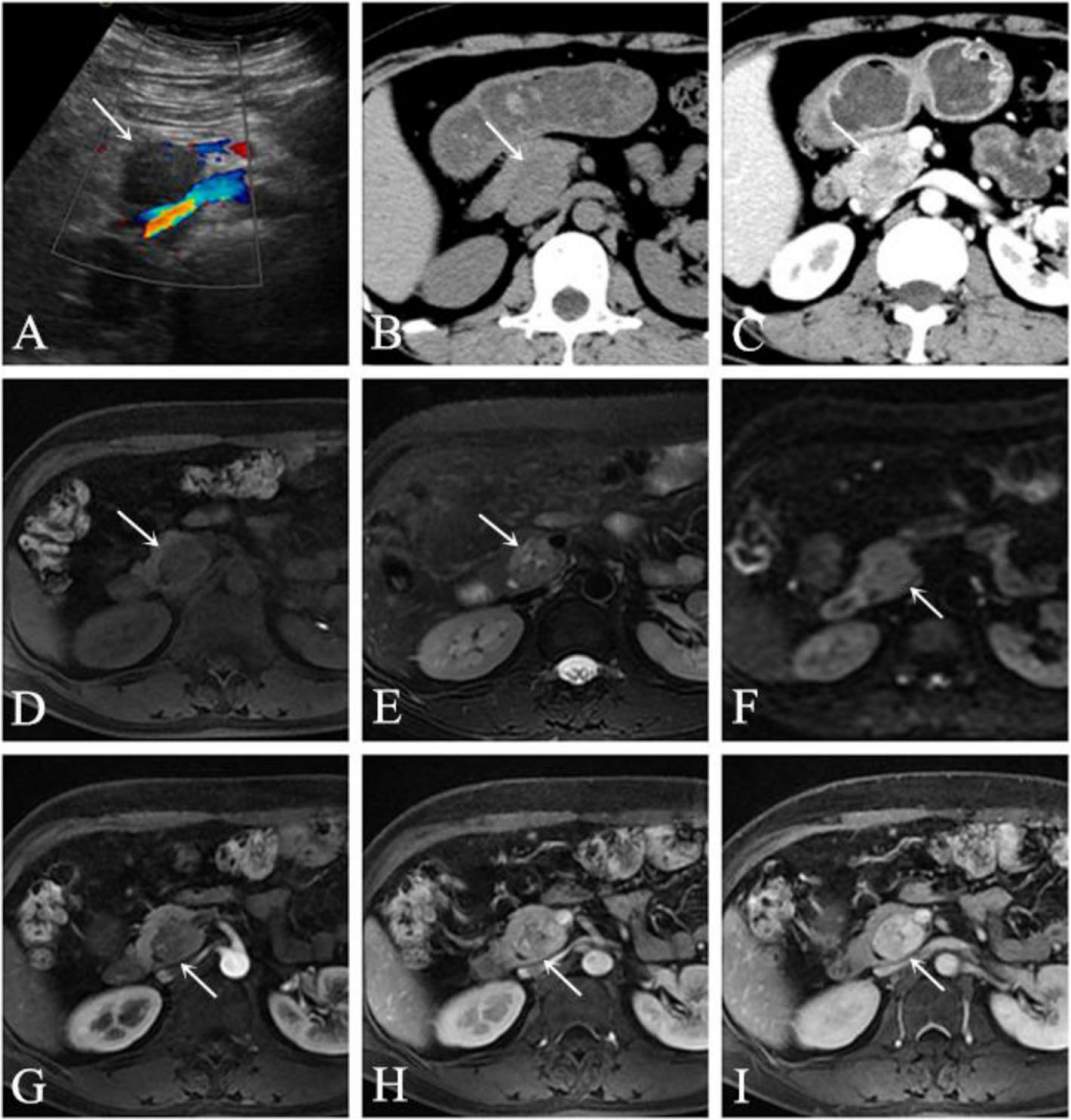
The lesion showed a hypoechoic mass in the pancreatic head on US (a). Axial CT of the abdomen (plain scan and venous phase) showing a 2.9-cm iso-density lesion in the pancreatic head that was well-defined after enhancement (b-c). Axial pancreatic MRI showing a solid lesion with several small cystic lesions, T1WI showed hypointensity (d), T2WI showed iso- to high-intensity (e), and DWI showed iso-intensity (F); the lesion had obvious progressive enhancement (g-i)
